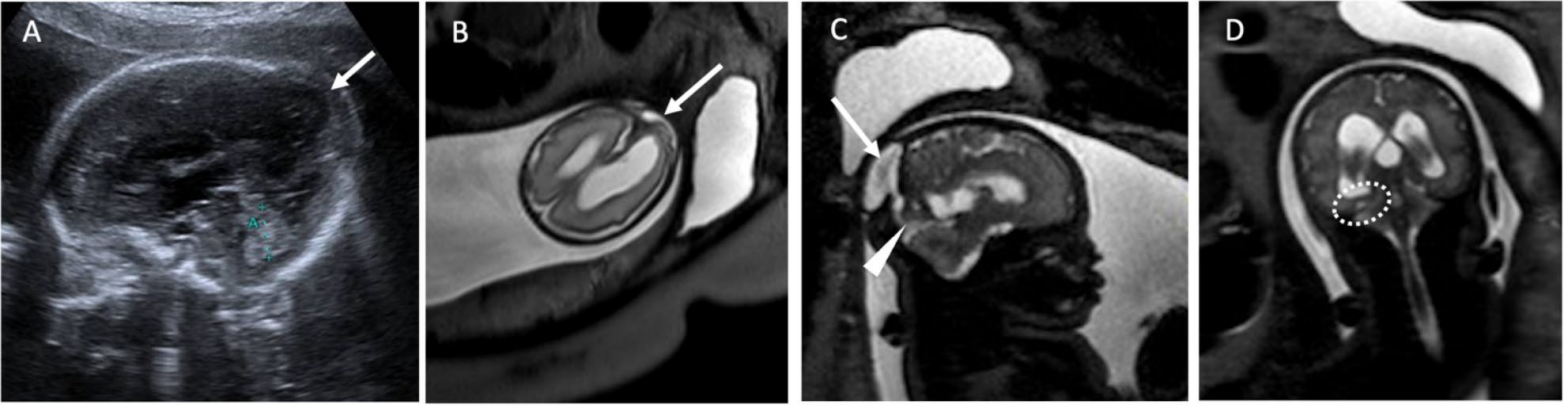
Twenty-six-week echography (A) and MRI (B axial view; C sagittal view; D coronal view) with the evidence of an encephalocele showing a parieto-occipital bone defect with a herniation of the cerebral hemisphere (white arrow). No anomaly of the posterior cerebral fossa was detected; however, there was a notable dehiscence of a large part of the cerebellar tent with (round in image D), potentially, a right sinus replaced by a falcoriel sinus corresponding to an accessory sinus rising towards the bone defect (point of arrow in image C). No major anomalies of cerebral organization except for small occipital subependymal heterotopias were described
